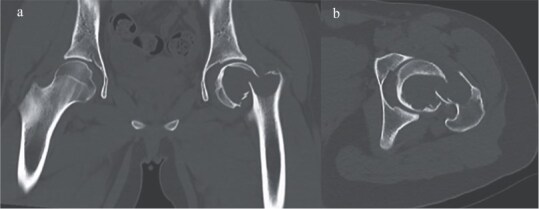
CT imaging revealed a Garden IV femoral neck fracture and a large radiolucent area occupying nearly the entire femoral head and extending into the neck. (a) Sagittal view and (b) coronal view.
